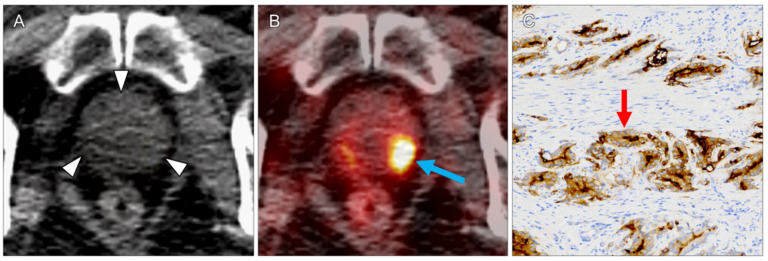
Incidental finding of a prostate carcinoma (lesion 3) in a patient who also had pulmonary and lymph node ccRCC metastases. On non-enhanced CT, the prostate appeared unremarkable ((A), arrowheads). PET/CT revealed high focal tracer uptake in the left peripheral zone ((B), arrow; SUVmax 9.3). In the biopsy-proven prostate carcinoma, strong PSMA expression on the cytoplasmatic membranes was seen ((C), arrow).
